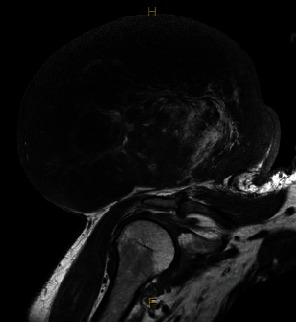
MRI of right shoulder: preradiotherapy treatment in the frontal plane.
